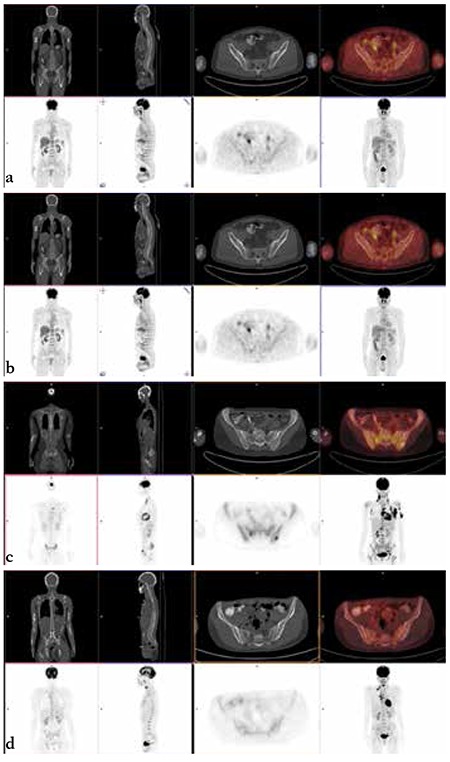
Positron emission tomography and computed tomography images. a) Aggressive non-Hodgkin’s lymphoma patient bone marrow involvement-positive in bone marrow biopsy and bone marrow involvement-negative in positron emission tomography and computed tomography imaging, b) Aggressive non-Hodgkin’s lymphoma patient bone marrow involvement-negative in bone marrow biopsy and bone marrow involvement-positive in positron emission tomography and computed tomography imaging, c) Hodgkin’s lymphoma patient bone marrow involvement-positive in bone marrow biopsy and positron emission tomography and computed tomography imaging together, d) Hodgkin’s lymphoma patient bone marrow involvement-negative in bone marrow biopsy and positron emission tomography and computed tomography imaging together.
